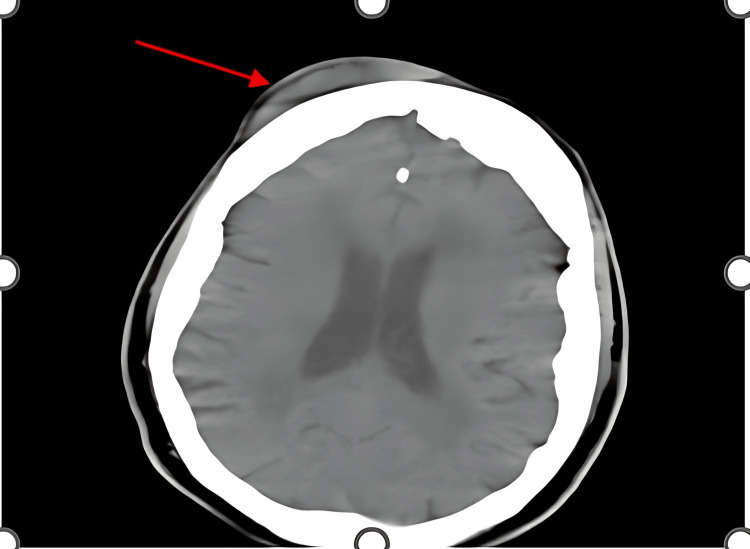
Axial CT without contrast scan of the head taken in 2020. The red arrow indicates a right frontal hematoma.
